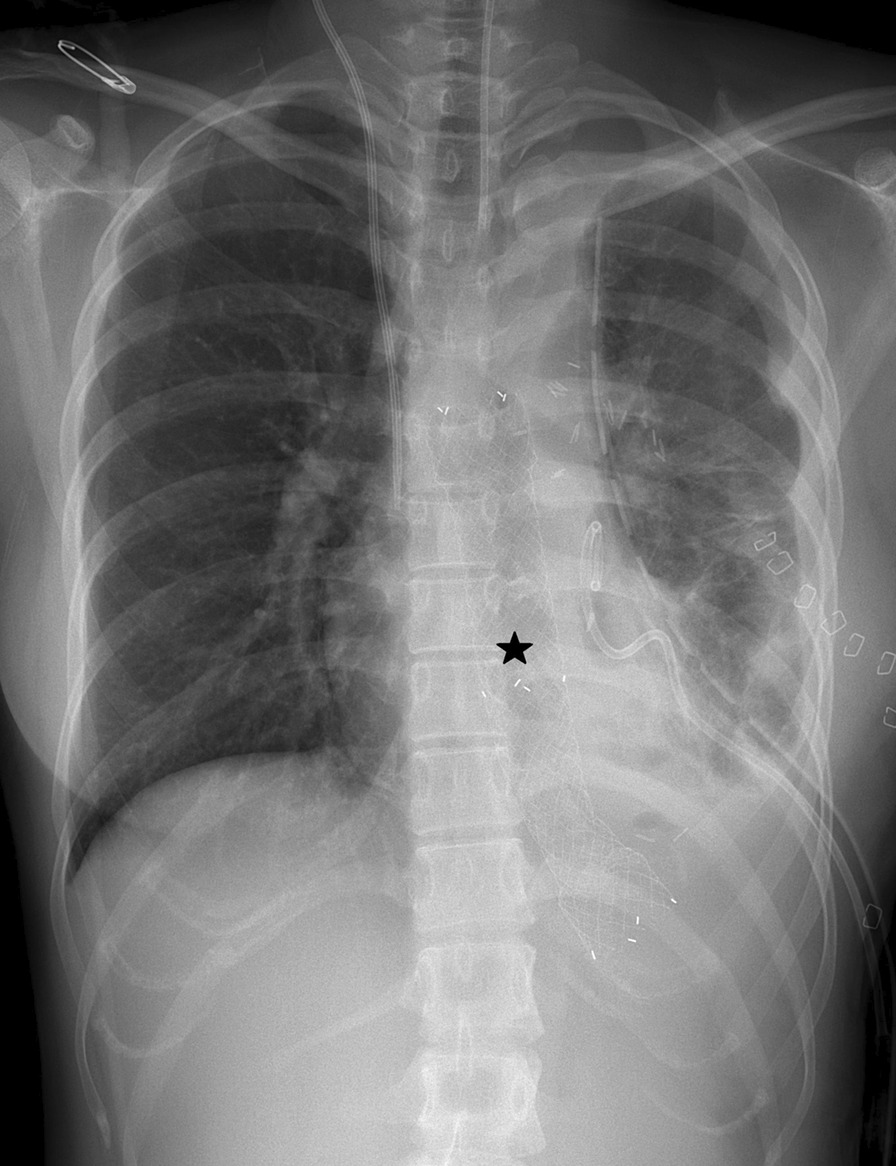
Esophageal stent observed on chest X-ray (*)
